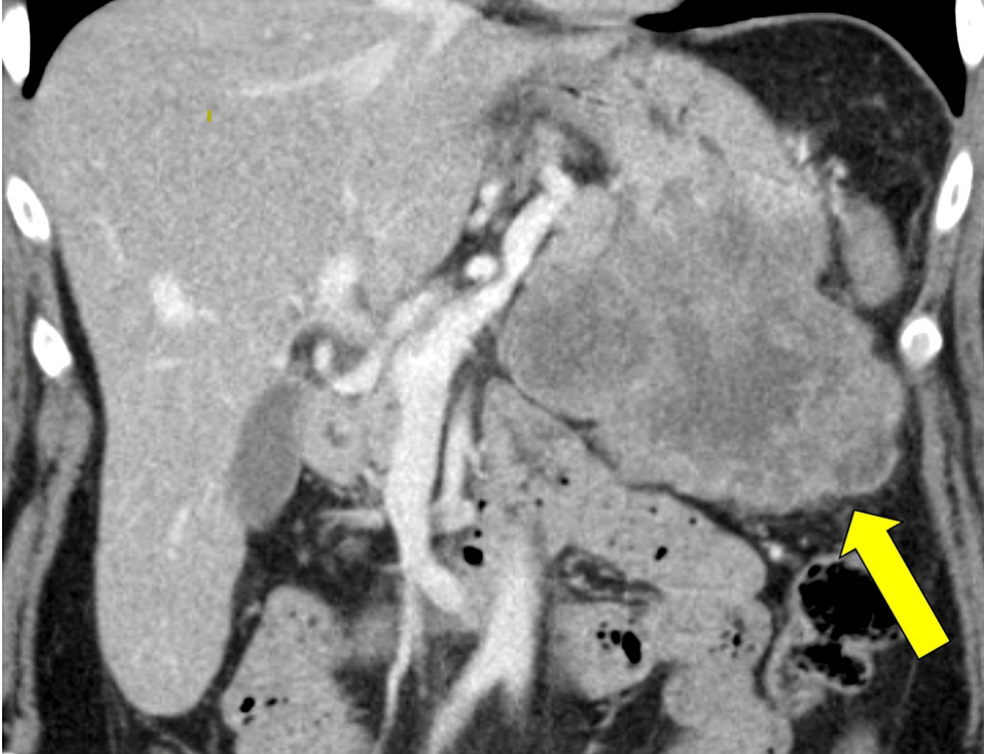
CT Abdomen of Primary Gastrointestinal Stromal Tumor (GIST) in Posterior Wall and Greater Curvature of Stomach
